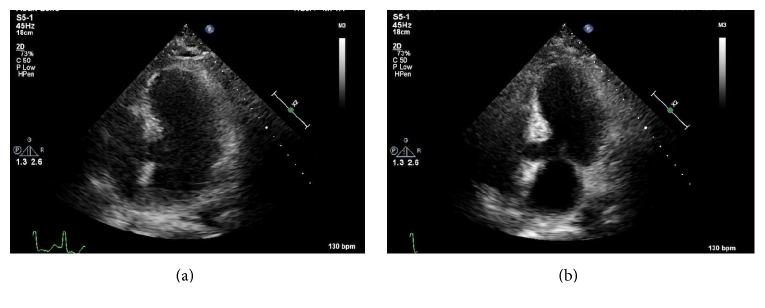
Echocardiographic evidence of classic takotsubo cardiomyopathy, the apical type. (a) represents diastole and (b) represents systole.
